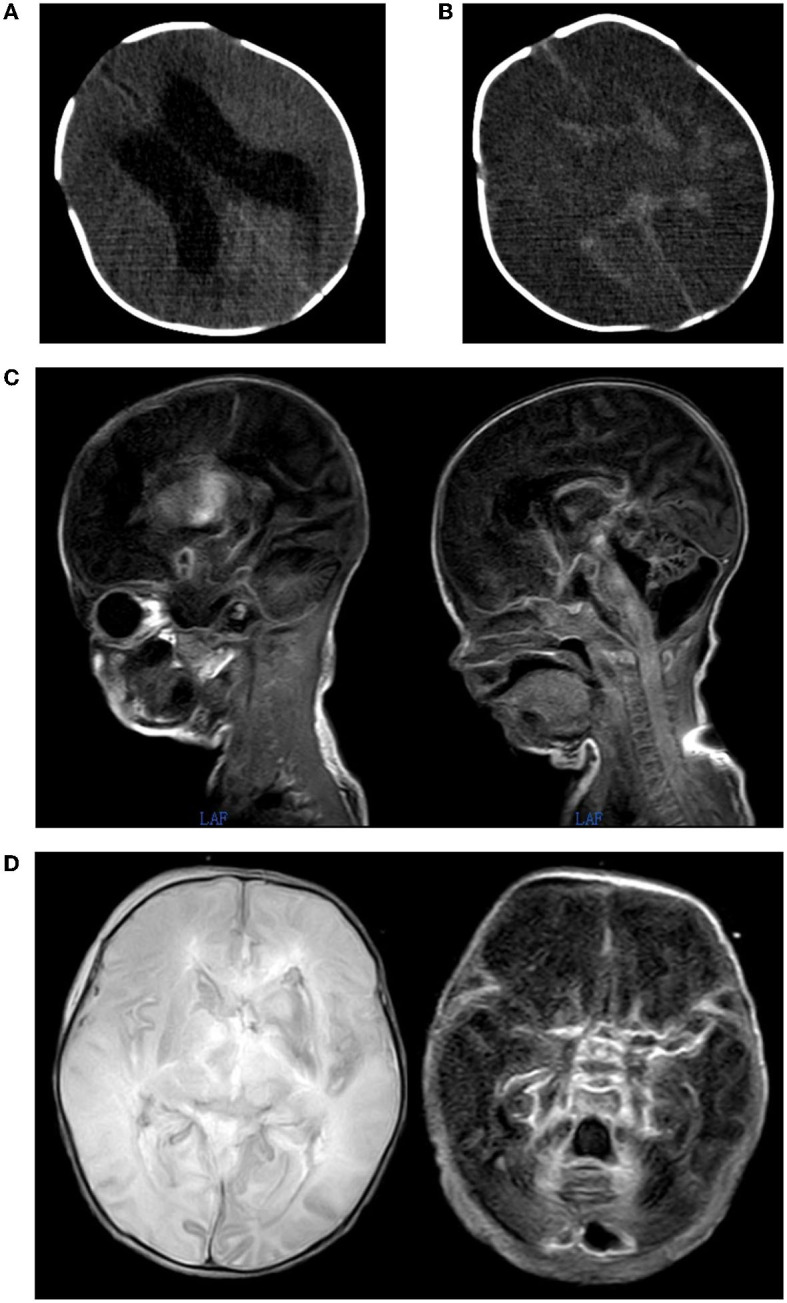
(A) On 2022.01.19th, head CT shows no obvious abnormal density lesions in the brain parenchyma, enlargement of the ventricular with lateral ventricular dilatation as the main feature, no displacement of midline structures and no widening of the sulcus pool. (B) On 2022.01.24th, head CT scan showed severe cerebral edema and significant shrinkage of the ventricular compared with before. (C, D) On 2022.01.26th, MRI scan of the head showed changes in the left ventricle after puncture drainage. Severe cerebral edema suggested the presence of ventricular and subarachnoid hemorrhage.
